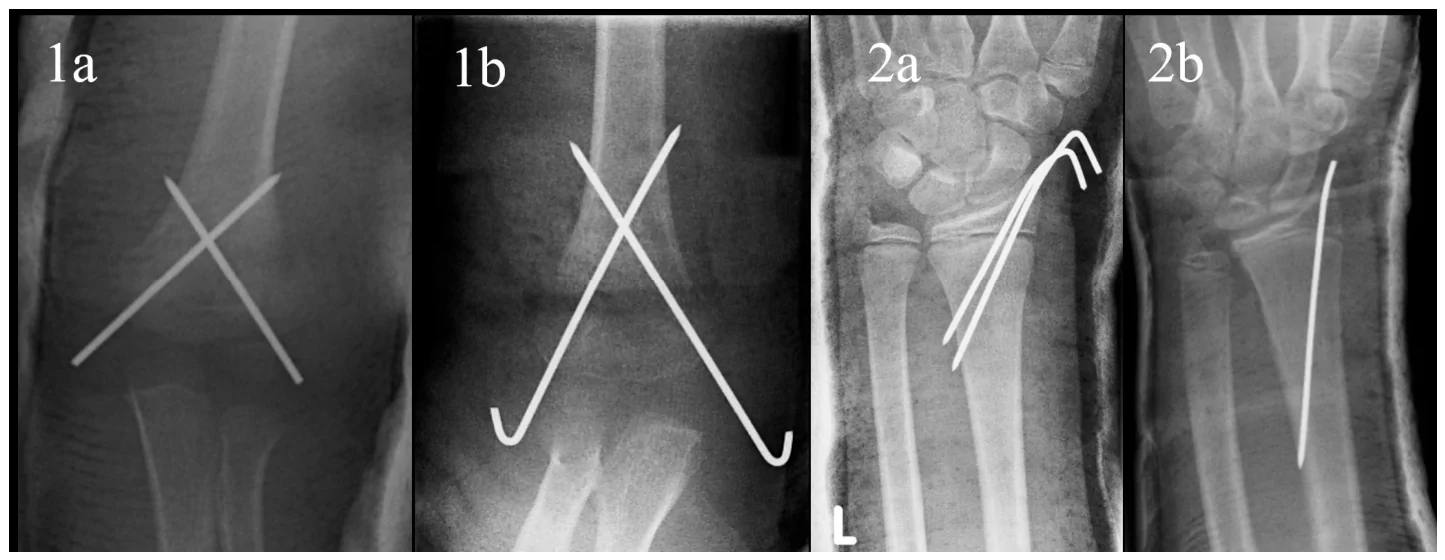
Radiographic examples of K-wire osteosyntheses: Buried K-wire osteosyntheses of the distal humerus (1a) and distal radius (2a) and unburied K-wire osteosyntheses of the distal humerus (1b) and distal radius (2b).
